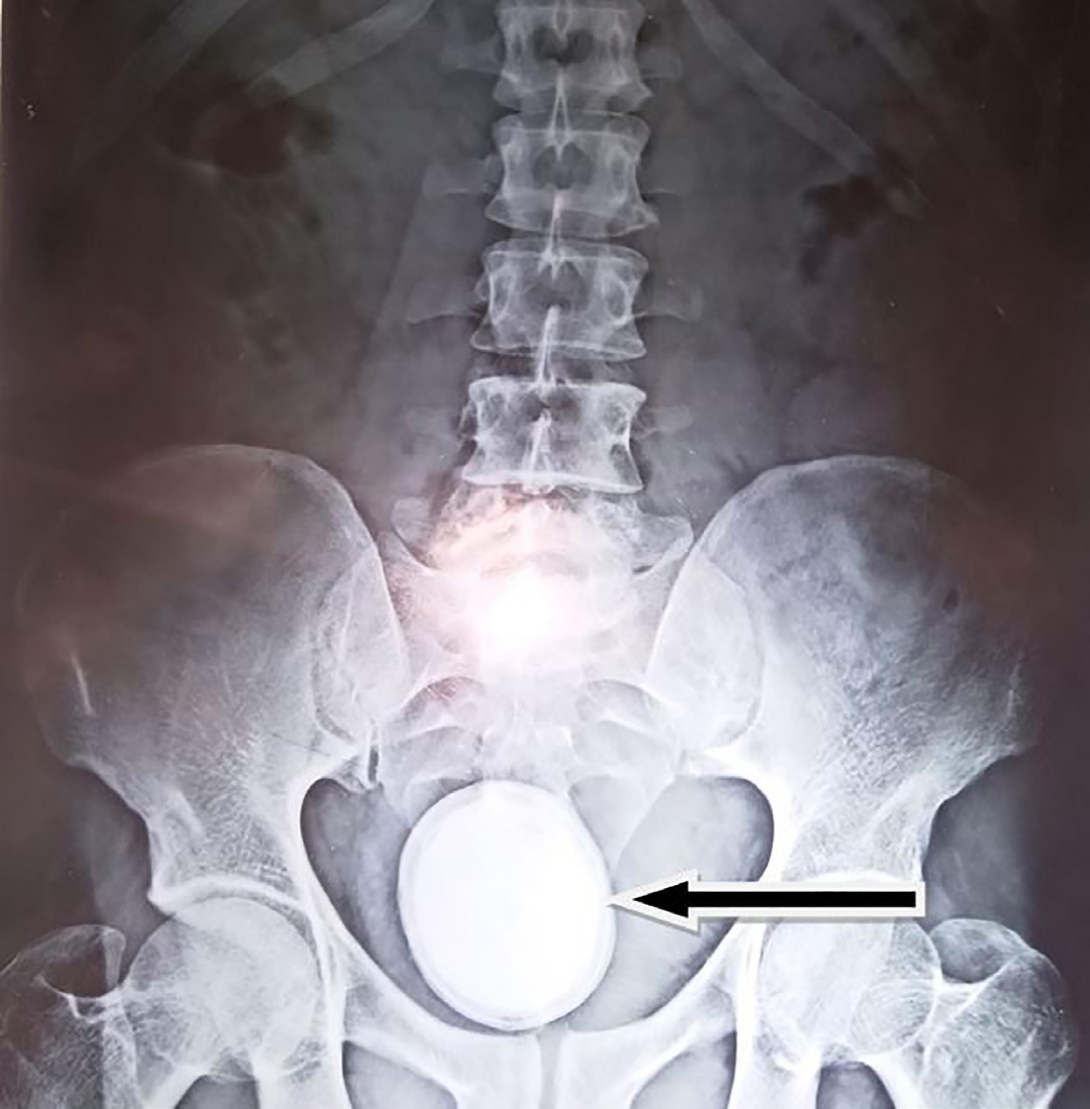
Radiograph of kidney, ureters and bladder showing a giant radiodensity overlying the bladder.
